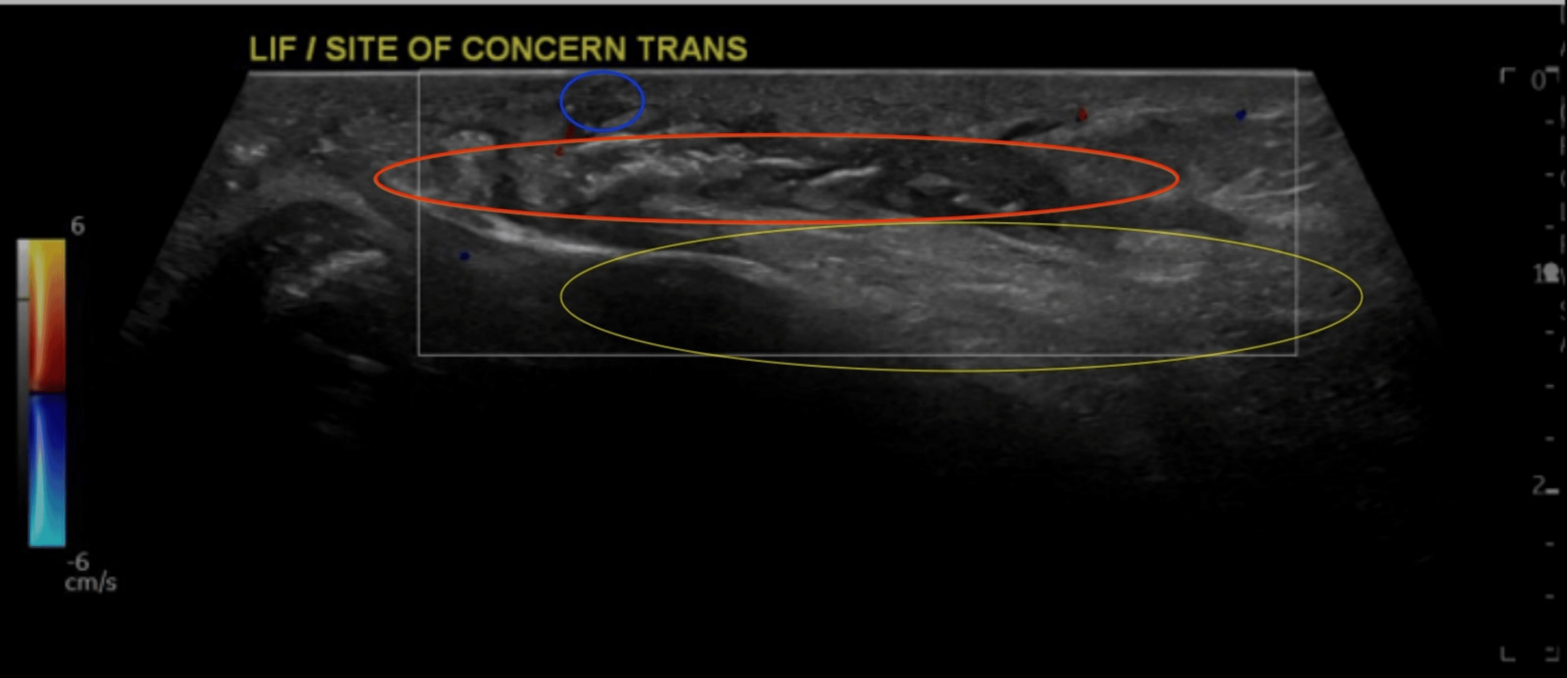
Ultrasound of the left hip surgical site revealed focal minimal superficial edema (yellow circle) associated with a fluid collection (red circle) tracking through a fine tract (blue circle) to the skin surface, without evidence of significant hypervascularity.
